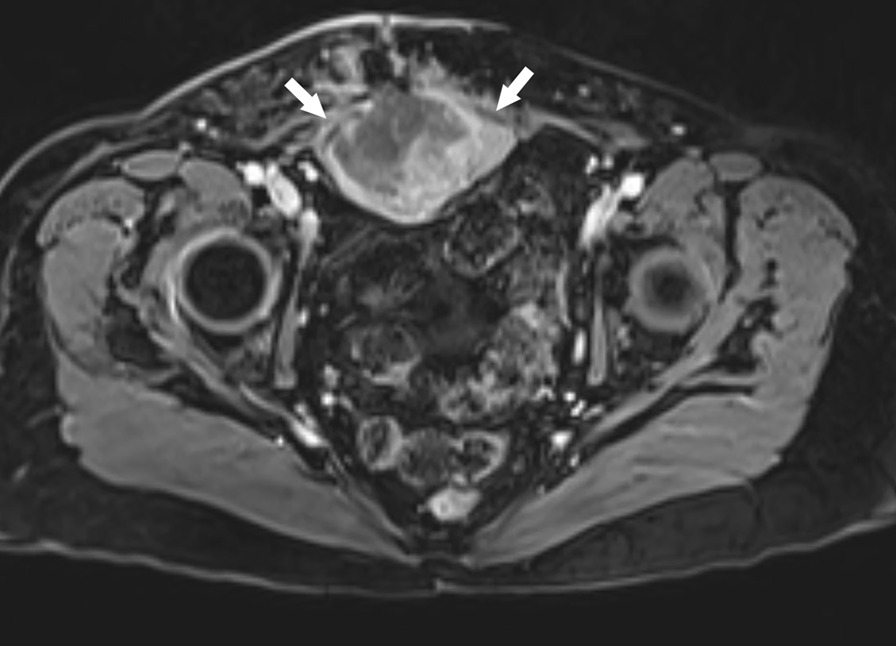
Axial T1 weighted post-contrast magnetic resonance imaging (MRI) demonstrating a large heterogeneously enhancing mass arising from the deep surface of the lower rectus abdominis muscle (arrows)
